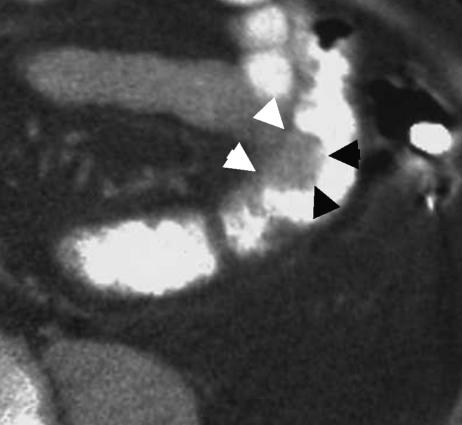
Preoperative CT scan showing a polypoid tumour of the descending colon extending into the lumen (black arrowheads). Tumour does not extend beyond the contour of the muscle coat indicating that this is an early T1/T2 tumour. Pathological staging was pT2.
